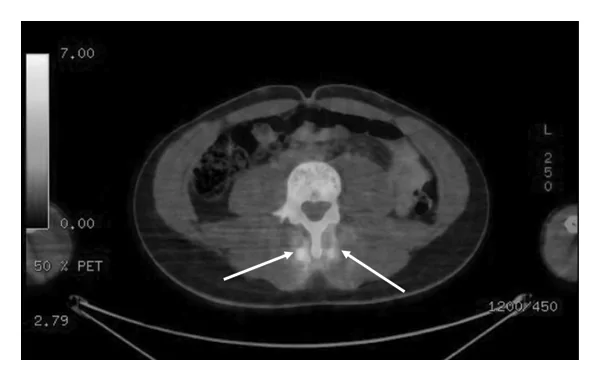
Transverse fluorodeoxyglucose positron emission tomography‐CT (PET‐CT) imaging of the lumbar region. The white arrow indicates peripheral enhancement of the bursa, associated with inflammation of the interspinous bursa between the third and fourth lumbar vertebrae (L3 and L4).
